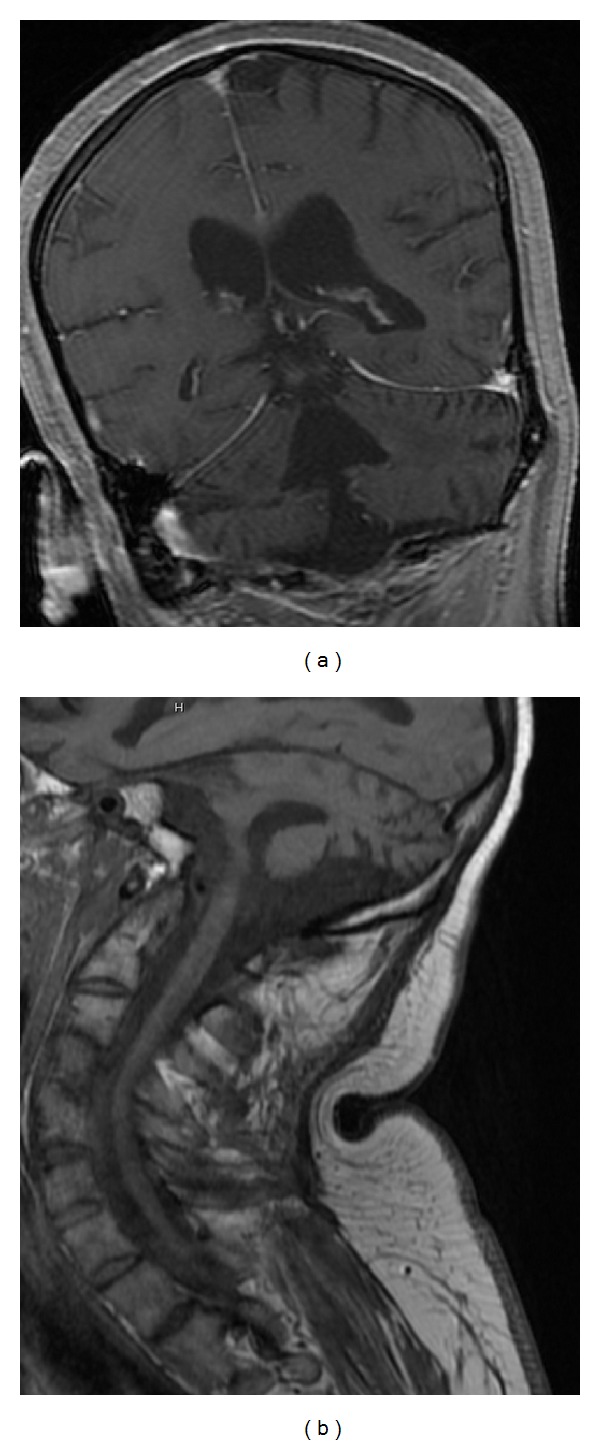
(a) and (b) (Seven months after initial MRI). MRI brain (a) showed marked atrophy of the brainstem, predominantly in the pons. MRI cervical spine (b) showed increased volume loss in the cervical cord on T-1 weighted image.
